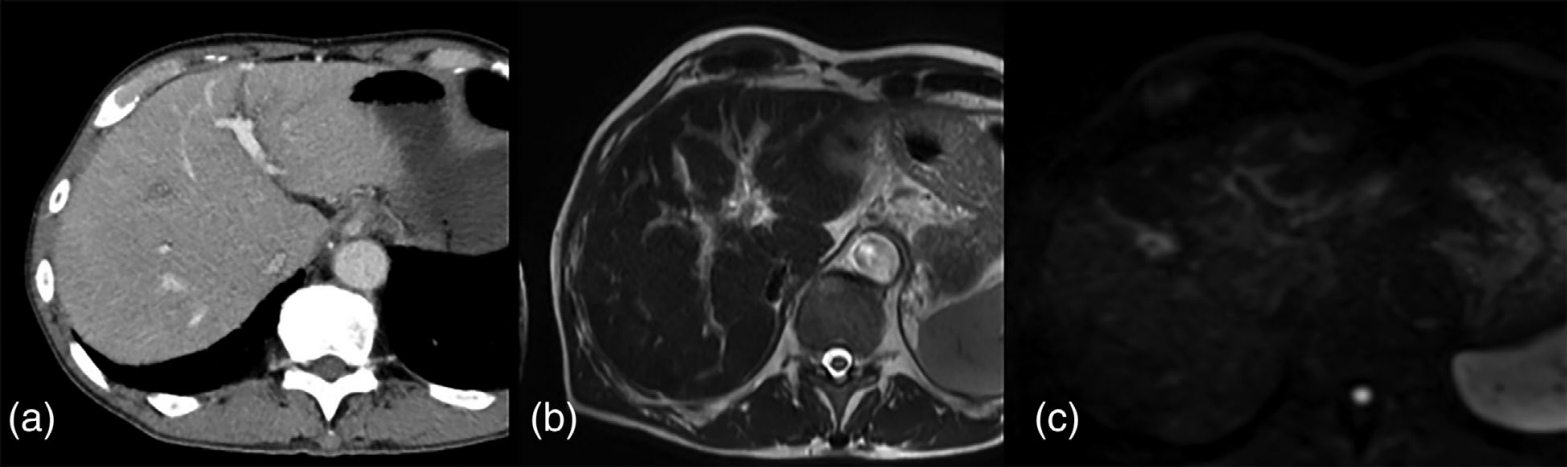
(a) The enhanced computed tomography (CT) image does not reveal a massive tumor, but low density in the periportal area can be detected. (b),(c) T_2_‐weighted and diffusion‐weight magnetic resonance imaging (MRI) shows high intensity at the periportal area
